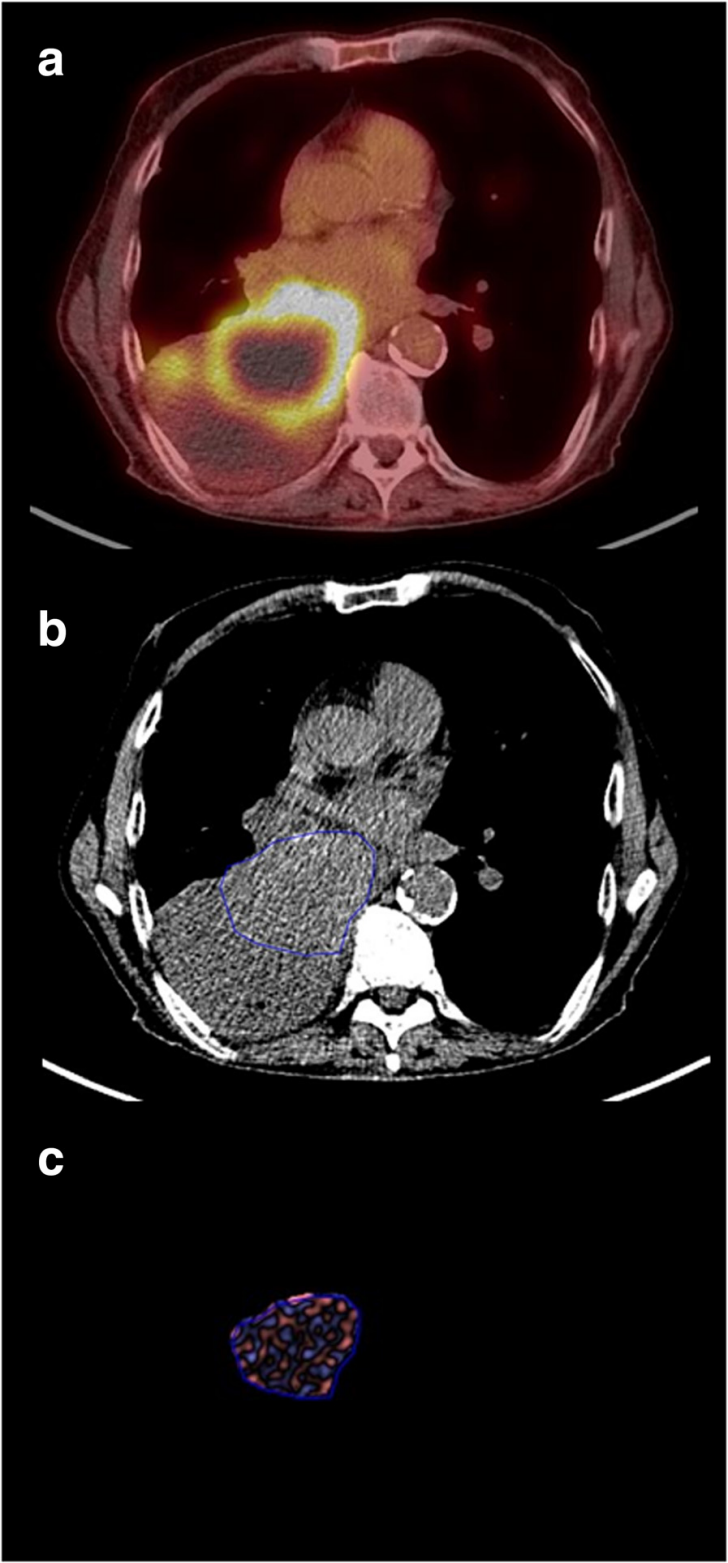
Right lower lobe NSCLC showing necrosis without cavitation (photopaenia on FDG-PET/CT) and adjacent pulmonary consolidation (a). Due to minimal contact with aerated lung, the tumour ROI has been constructed manually (b), using the fused PET/CT image and narrow windows for guidance. The area of necrosis without cavitation is included in the the ROI and the final filtered tumour image (c)
